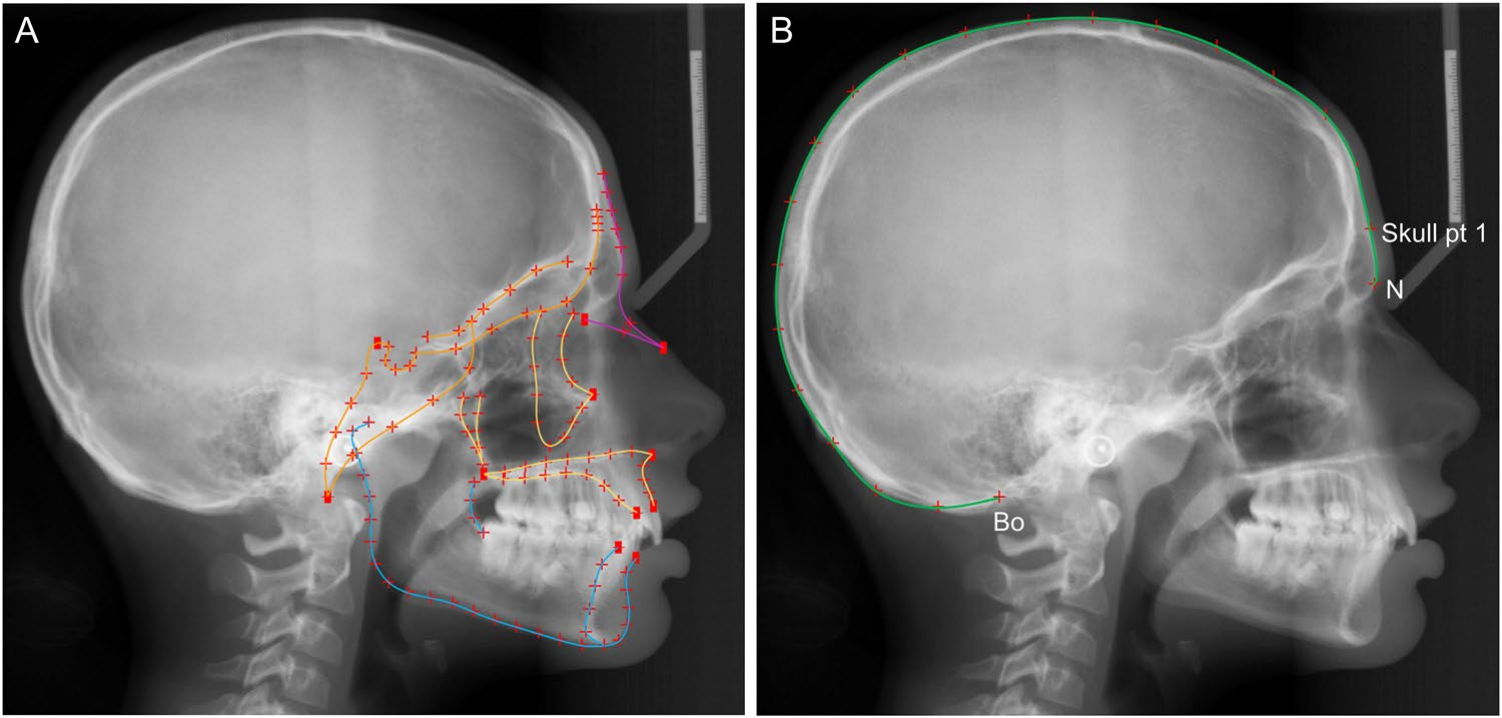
Landmarks used to capture craniofacial morphology. (A) Digitization of the craniofacial complex (n = 808) with 15 curves, which included 116 semilandmarks (red crosses), and 11 fixed landmarks (red rectangles). Orange color represents the structures of the cranial base, yellow the maxillary structures, blue the mandibular structures, and all lines together the whole configuration. (B) Additional curve digitized in radiographs (n = 112) that also included the whole cranium (green color). This curve started at point N and ended at Bolton (Bo) point. 20 semilandmarks, initially spread equidistantly on this curve were used to capture cranial morphology, starting from a point located at the 5% of the N-Bo distance (Skull pt 1) and ending at Bo point.
